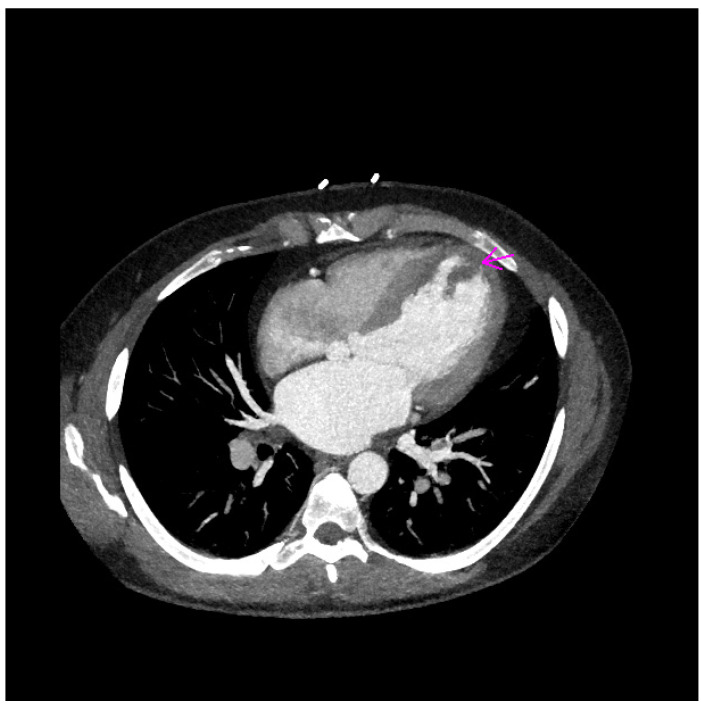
CT finding of first thrombus in the area of left ventricular apex (purple arrow).
